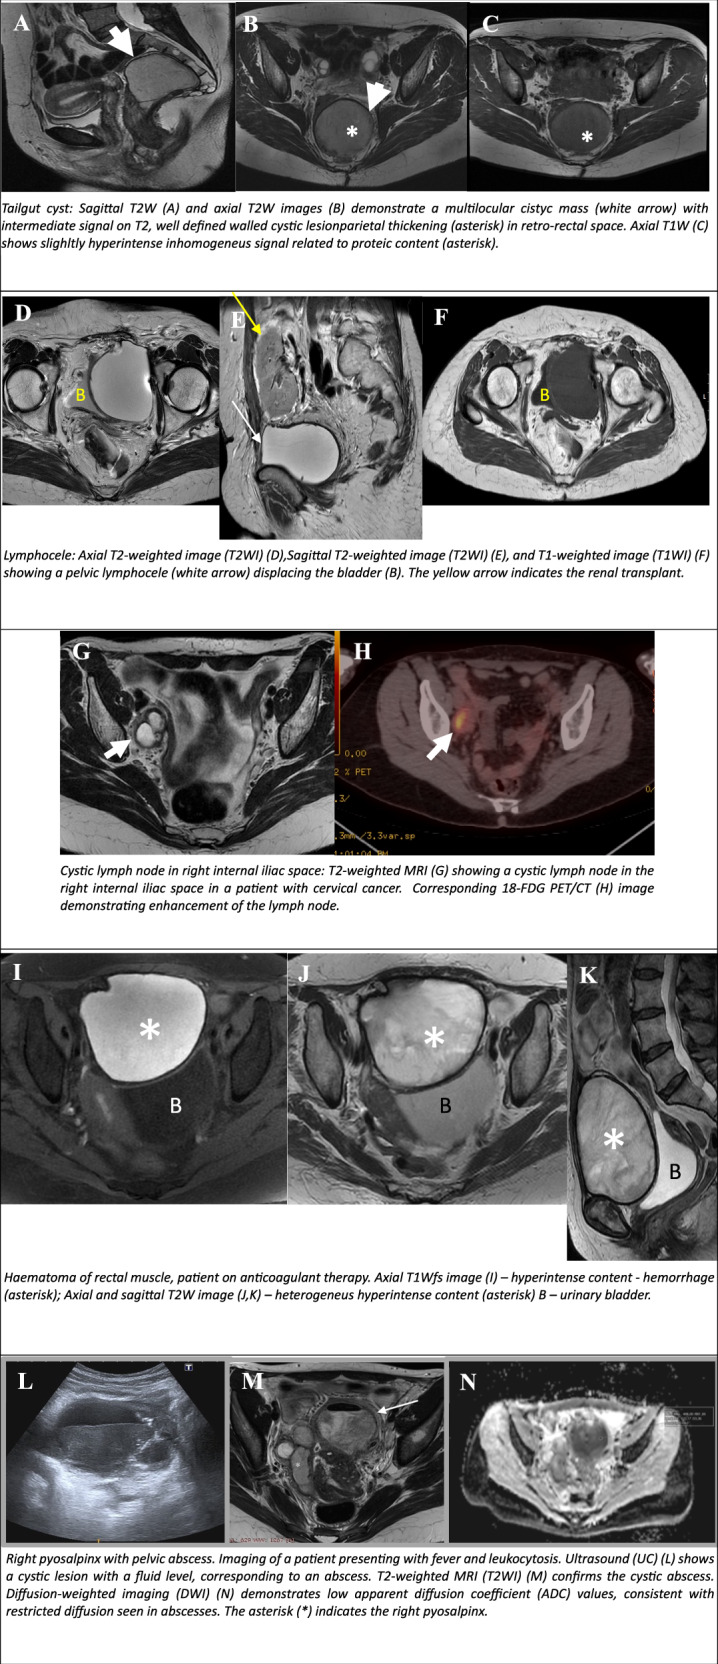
Non-ovarian cystic female pelvic lesions. Tailgut cyst: sagittal T2W (A) and axial T2W images (B) demonstrate a multilocular cystic mass (white arrow) with intermediate signal on T2, a well-defined walled cystic lesion with parietal thickening (asterisk) in the retro-rectal space. Axial T1W (C) shows a slightly hyperintense inhomogeneous signal related to proteic content (asterisk). Lymphocele: axial T2WI (T2WI) (D), sagittal T2WI (T2WI) (E), and T1-weighted image (T1WI) (F) showing a pelvic lymphocele (white arrow) displacing the bladder (B). The yellow arrow indicates the renal transplant. Cystic lymph node in right internal iliac space: T2-weighted MRI (G) showing a cystic lymph node in the right internal iliac space in a patient with cervical cancer. Corresponding 18-FDG PET/CT (H) image demonstrating enhancement of the lymph node. Haematoma of the rectal muscle, patient on anticoagulant therapy. Axial T1Wfs image (I)—hyperintense content—haemorrhage (asterisk); axial and sagittal T2W image (J, K)—heterogeneous hyperintense content (asterisk) B—urinary bladder. Right pyosalpinx with pelvic abscess. Imaging of a patient presenting with fever and leukocytosis. Ultrasound (UC) (L) shows a cystic lesion with a fluid level, corresponding to an abscess. T2-weighted MRI (T2WI) (M) confirms the cystic abscess. Diffusion-weighted imaging (DWI) (N) demonstrates low apparent diffusion coefficient (ADC) values, consistent with restricted diffusion seen in abscesses. The asterisk (*) indicates the right pyosalpinx
